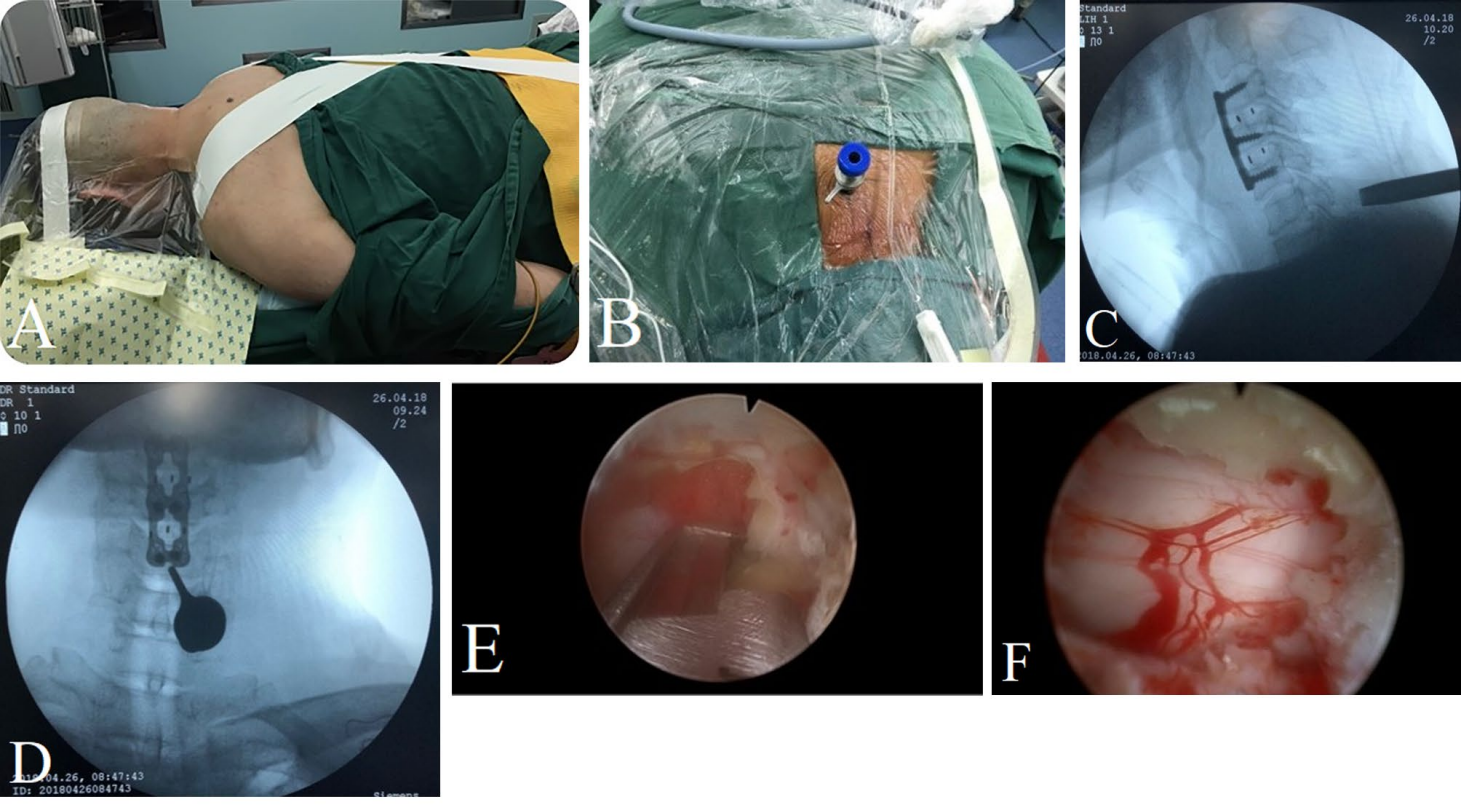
Posterior endoscopic decompression procedure. (A) Patient was placed in the prone position under general anesthesia. (B) The working channel was placed at the corresponding level with an 8–10 mm incision. (C and D) Intraoperative lateral and anterior X-ray radiograph of cervical vertebrae confirmed position of the working channel. (E) Decompression of dorsal elements by a rongeur. (F) Directly visual decompression of spinal cord
